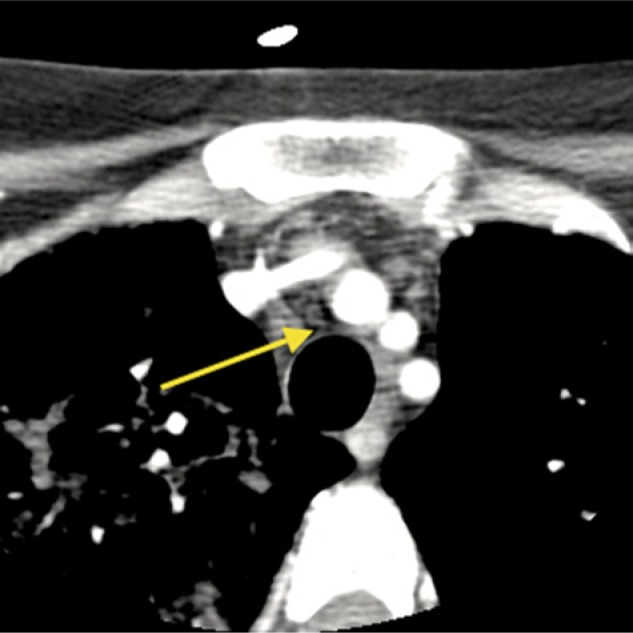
Computed tomography scan of the neck: The arrow indicates a soft tissue between the trachea and innominate artery.
